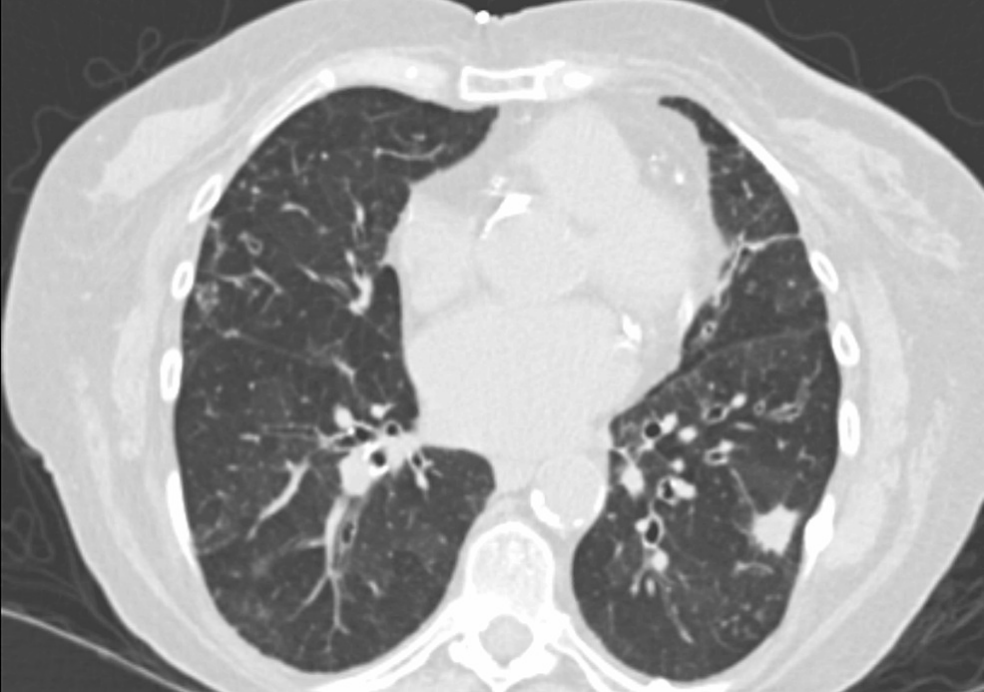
A non-contrast CT scan of the chest demonstrated patchy ground-glass opacities, bronchiectasis of moderate severity, and a left lower lobe nodule.
